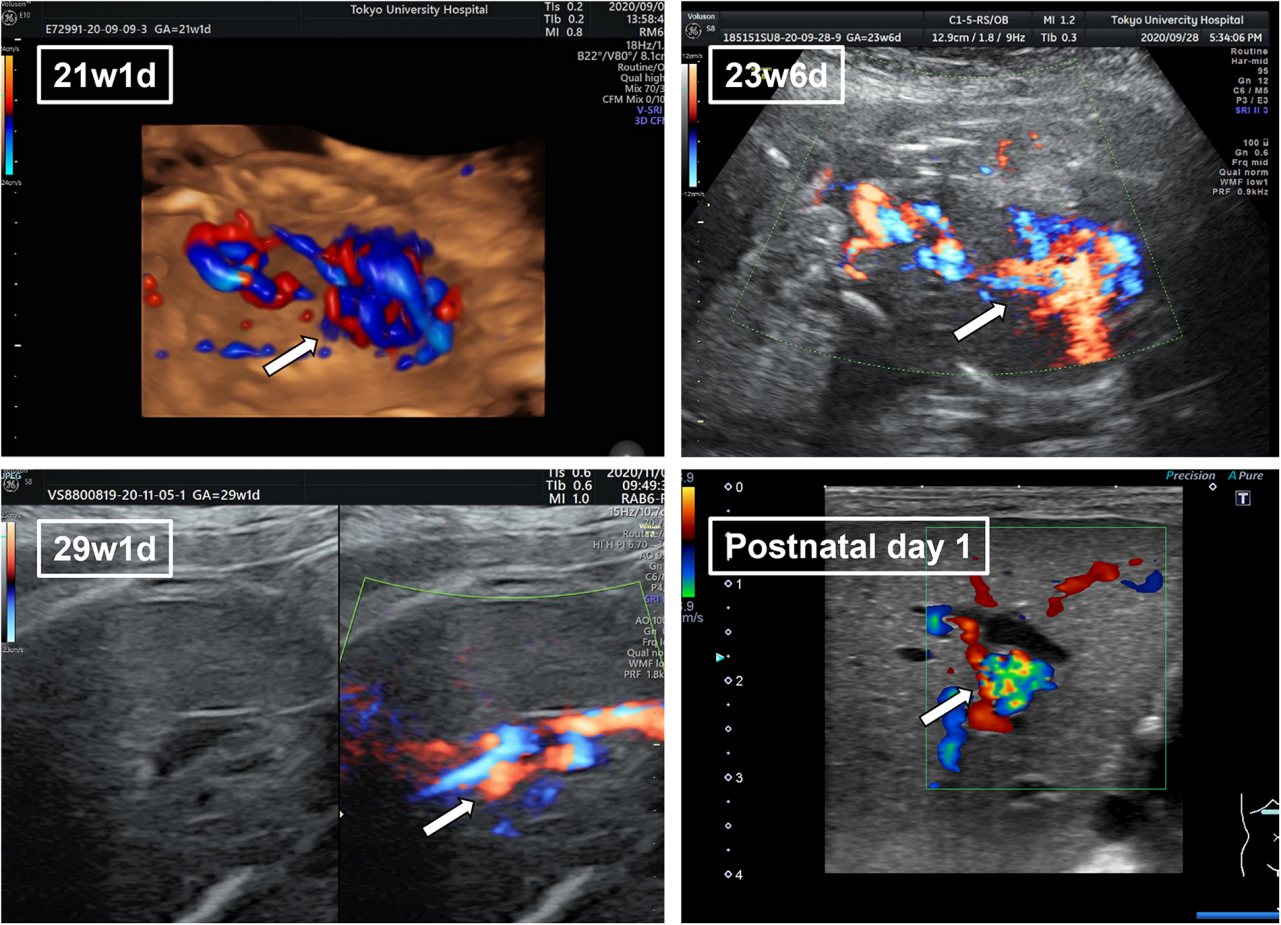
The fetal ultrasonography of umbilical vein varix (UVV) in the perinatal period. Fetal ultrasonography revealed a 17 mm × 10 mm UVV near the hepatic hilum (arrow). Dilation of the umbilical vein remained the same throughout the perinatal period.
